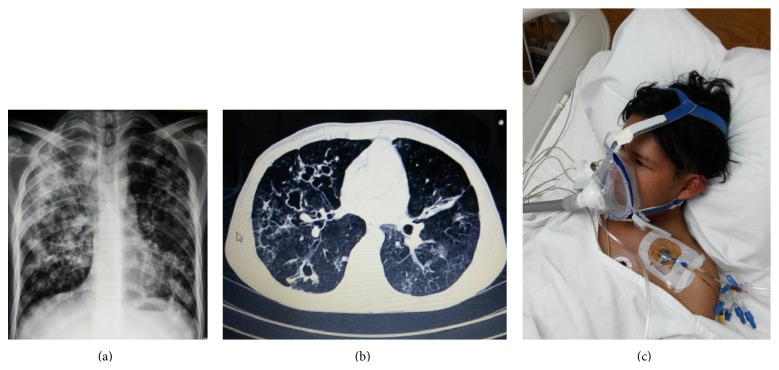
(a) Chest radiograph showing extensive multifocal consolidation and cavitation predominantly in the right upper lobe. (b) Computed tomography (CT) image scan obtained 5 weeks later shows the persistence of some caverns, nodules, and linear opacities but a significant improvement in areas of consolidation. (c) An oronasal mask was used to minimize air leakage and improve tolerance for noninvasive ventilation. Health personnel should not overlook the risk of tuberculosis transmission associated with short distances exposures.
